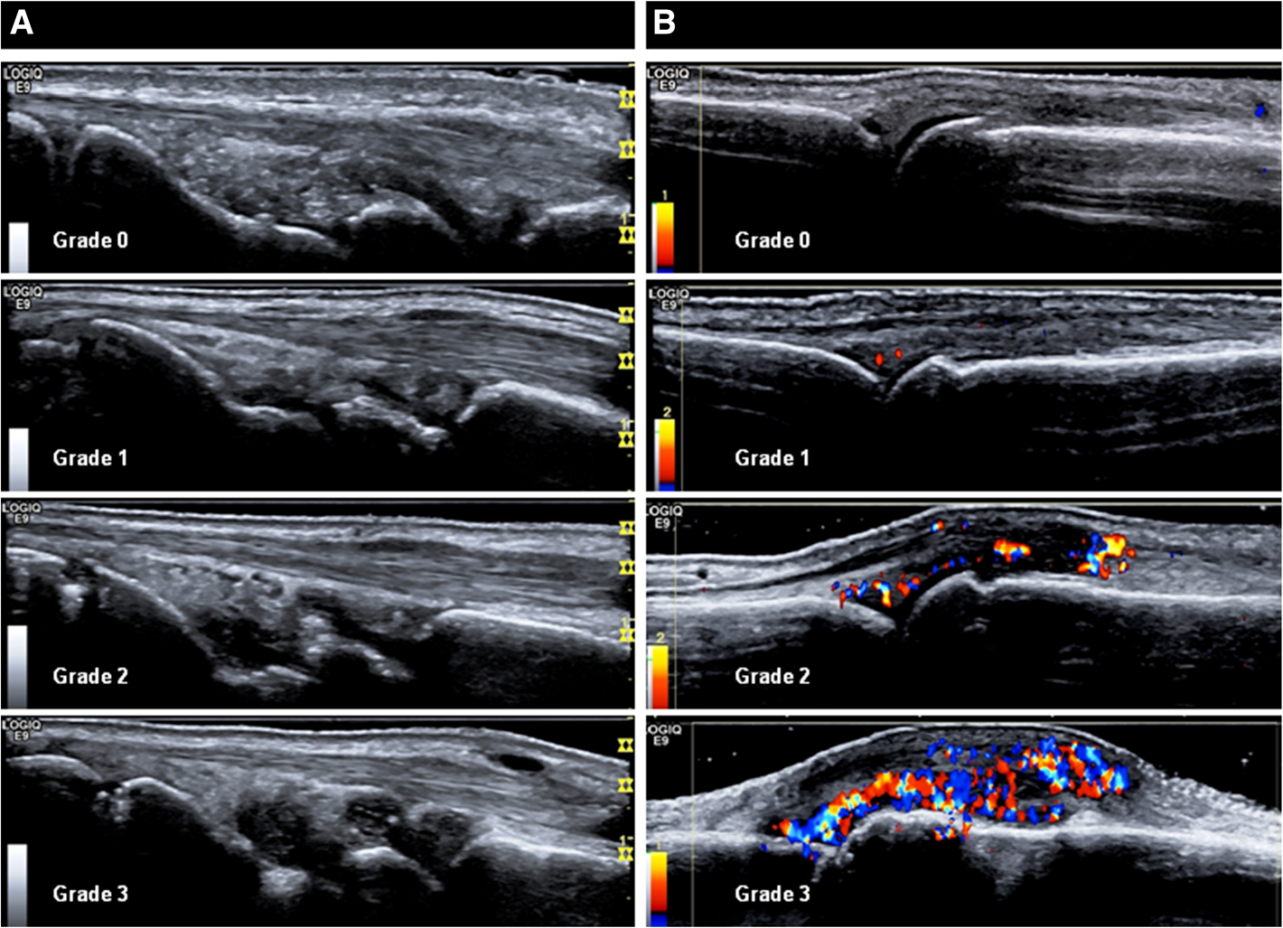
Semiquantitative ultrasound score in B-mode at the wrist joint in the central dorsal longitudinal position (A) and color Doppler semiquantitative ultrasound at the metacarpophalangeal (MCP) joints in the dorsal longitudinal position (B). Cut off for positive musculoskeletal ultrasound finding was grade one in both B-mode and color Doppler ultrasound in this study.
